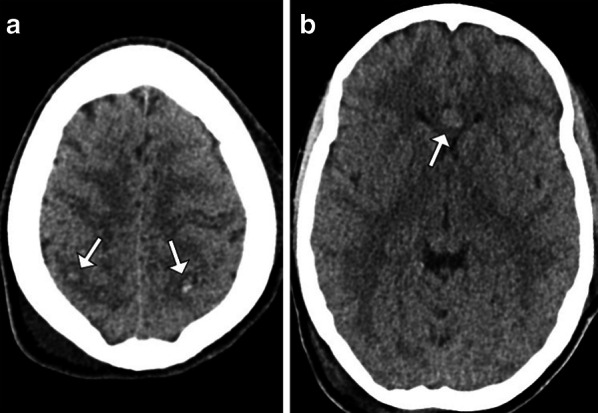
A case showing microhemorrhages as seen on CT. Axial native CT scan images at the level of the centrum semiovale and the basal ganglia of a 16-year-old polytraumatised female patient acquired on postoperative day 11 after hospital admission. Multiple subcortical punctiform hyperdensities presumably representing microhemorrhages are seen bilaterally in the subcortical white matter (a), and a larger hyperdensity is seen in the genu of the corpus callosum (b)
